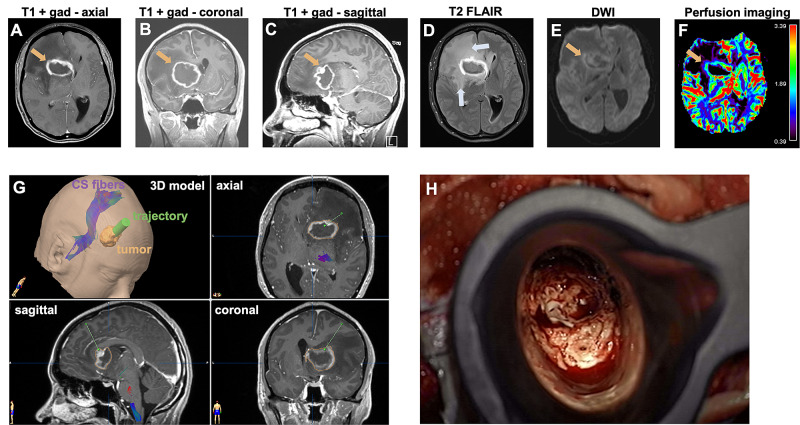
Preoperative imaging and surgical planning A, B, C: Preoperative T1-weighted MRI images enhanced with gadolinium show the ring-enhancing tumor (arrow) in the right basal ganglia. D: Axial T2 fluid-attenuated inversion recovery (FLAIR) image shows the peritumoral vasogenic edema (arrows). E: Axial diffusion-weighted image (DWI) shows only mild diffusion restriction (arrow). F: Axial perfusion imaging shows elevated relative cerebral blood flow (rCBV) along the enhancing rim of the tumor (arrow). G: Preoperative surgical planning on the BrainLab neuronavigation platform. The trajectory for placement of a tubular retractor is shown in green. Tractography was used to generate the corticospinal (CS) fiber tract. H: Intraoperative photograph showing the lesion visualized through the tubular retractor.
